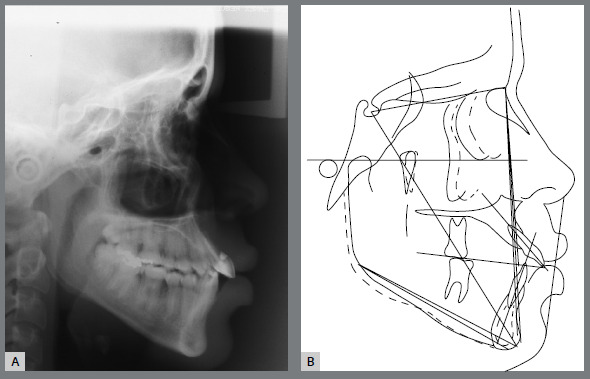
Pretreatment lateral cephalometric radiograph (A) and cephalometric tracing (B).
